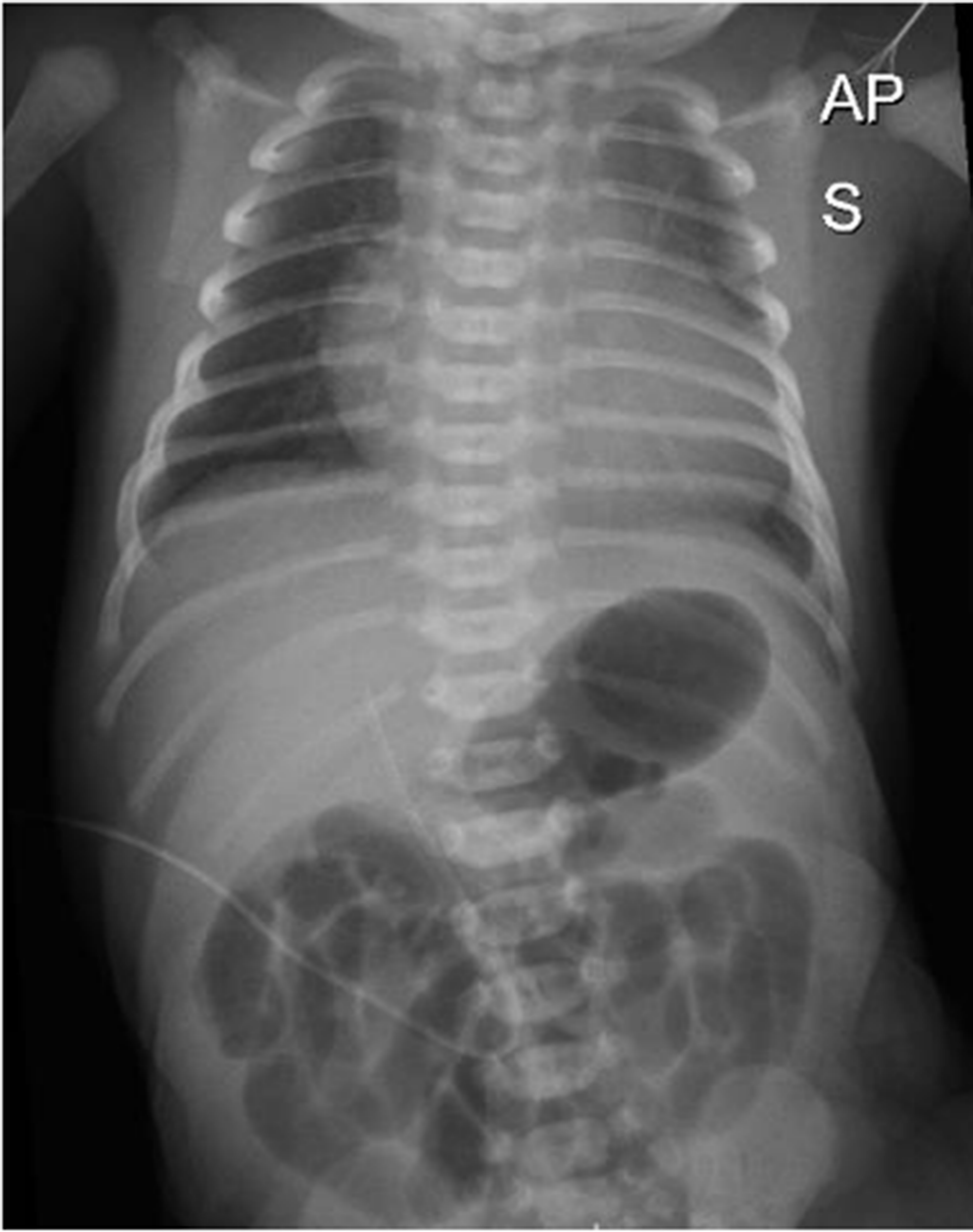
Chest X-Ray confirming severe cardiomegaly
